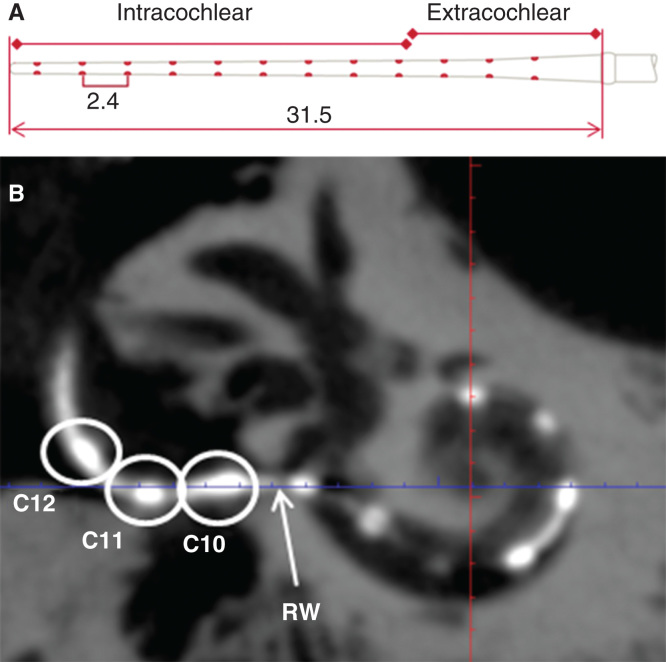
Measurement of full insertion depth of the electrode. A standard electrode array with the measures (mm) is shown in (A). On the cone-beam computed tomography (CBCT) image (B), three contacts (C10–C12) were outside the round window (RW) and the intracochlear measures started from the RW.
